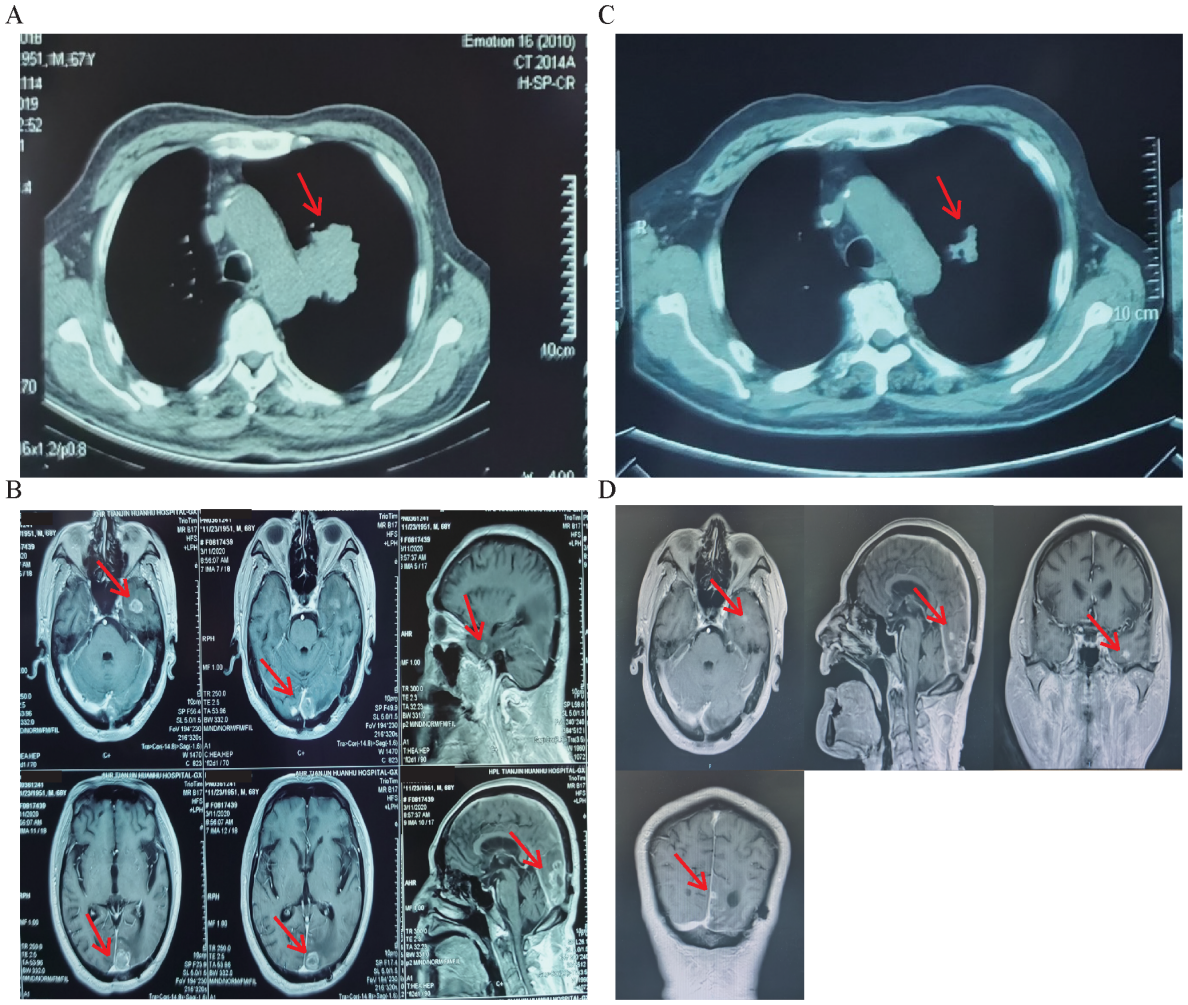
Chest computed tomography (CT) and head magnetic resonance imaging (MRI) results of case 2. (A, B) Chest CT and MRI results before immunotherapy combined with anti-angiogenic therapy. (C, D) Chest CT and MRI results after three cycles of immunotherapy combined with anti-angiogenic therapy. Arrows point to the location of the lesion.
